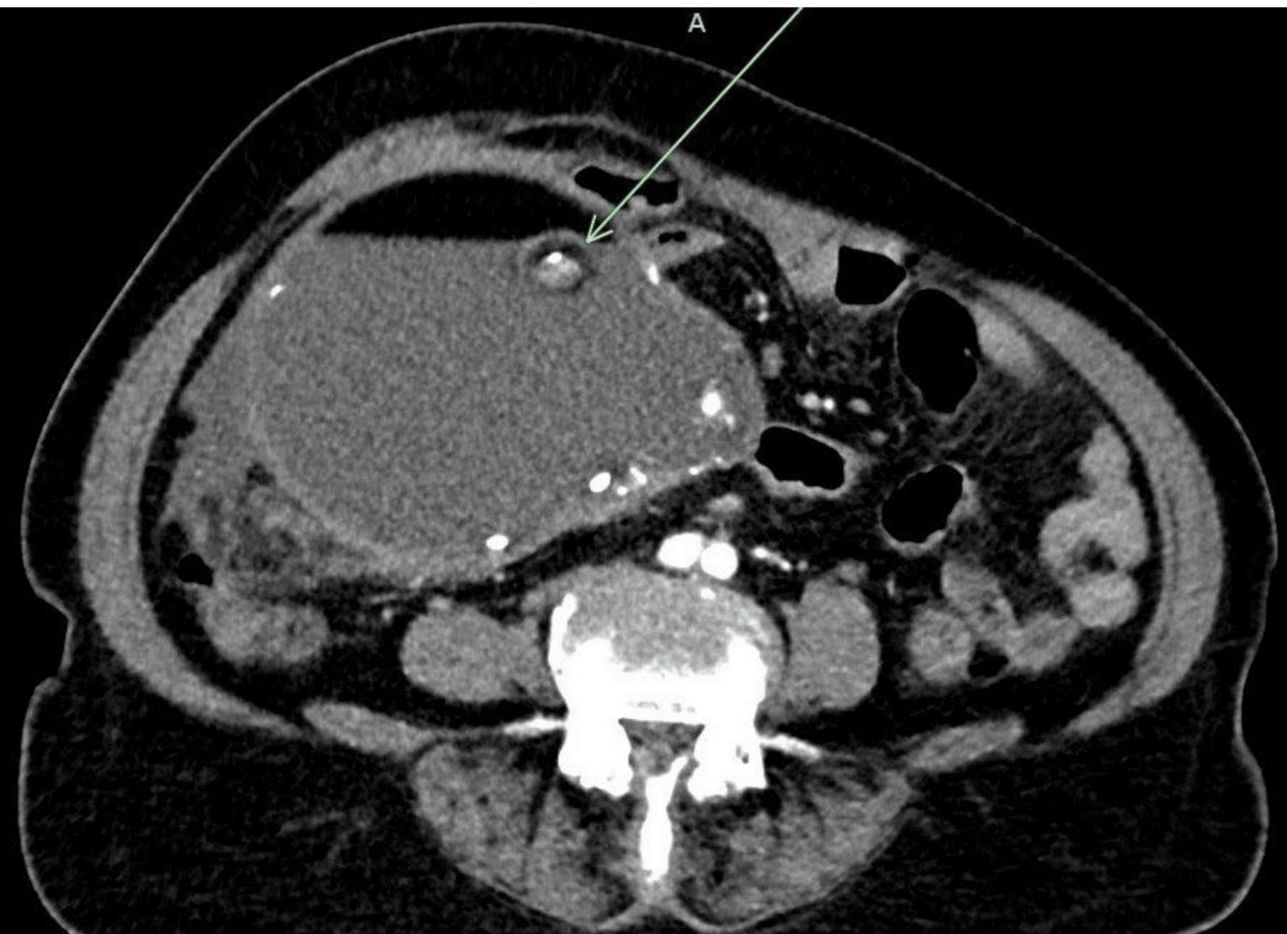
Axial contrast-enhanced CT image of the pelvis Axial contrast-enhanced CT image of the pelvis demonstrating a large, multiloculated cystic mass arising from the right adnexa, consistent with an ovarian teratoma with malignant transformation. The lesion demonstrates jagged internal density with areas of focal soft tissue enhancement. The green arrow delineates an area of capsular disruption, or rupture into the tumor, which was one of the reasons for assigning it FIGO (International Federation of Gynecology and Obstetrics) Stage IIIB (due to capsular disruption and mesoappendiceal involvement). The bowel loops and bladder are displaced due to mass effect; there are no gross ascites or peritoneal implants noted at this level.
